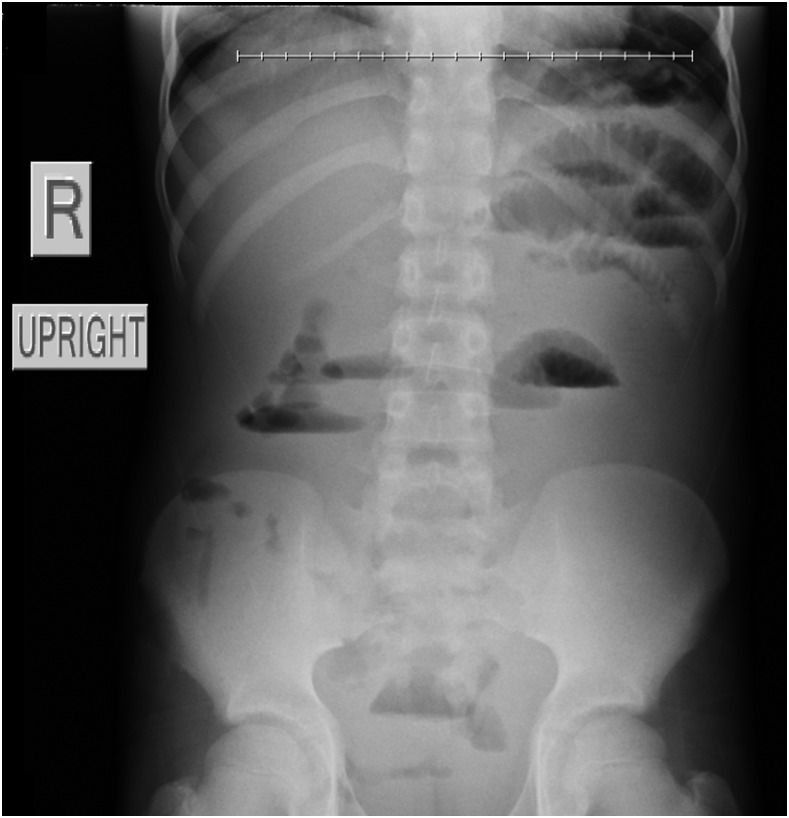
Abdominal X-ray demonstrating small bowel obstruction.
